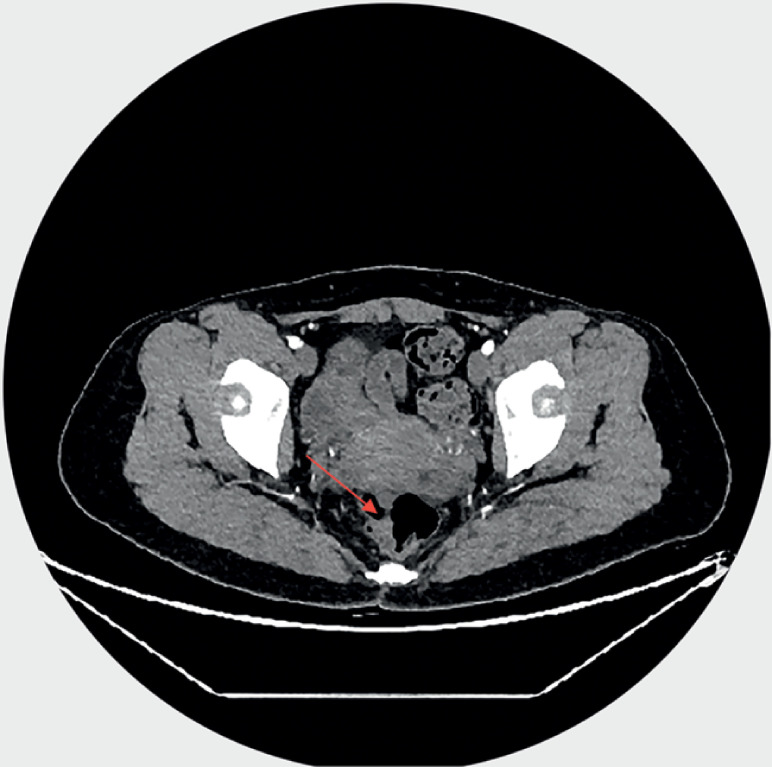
Preoperative abdominal contrast-enhanced computed tomography showing a lesion (red arrow) in the upper rectum.
